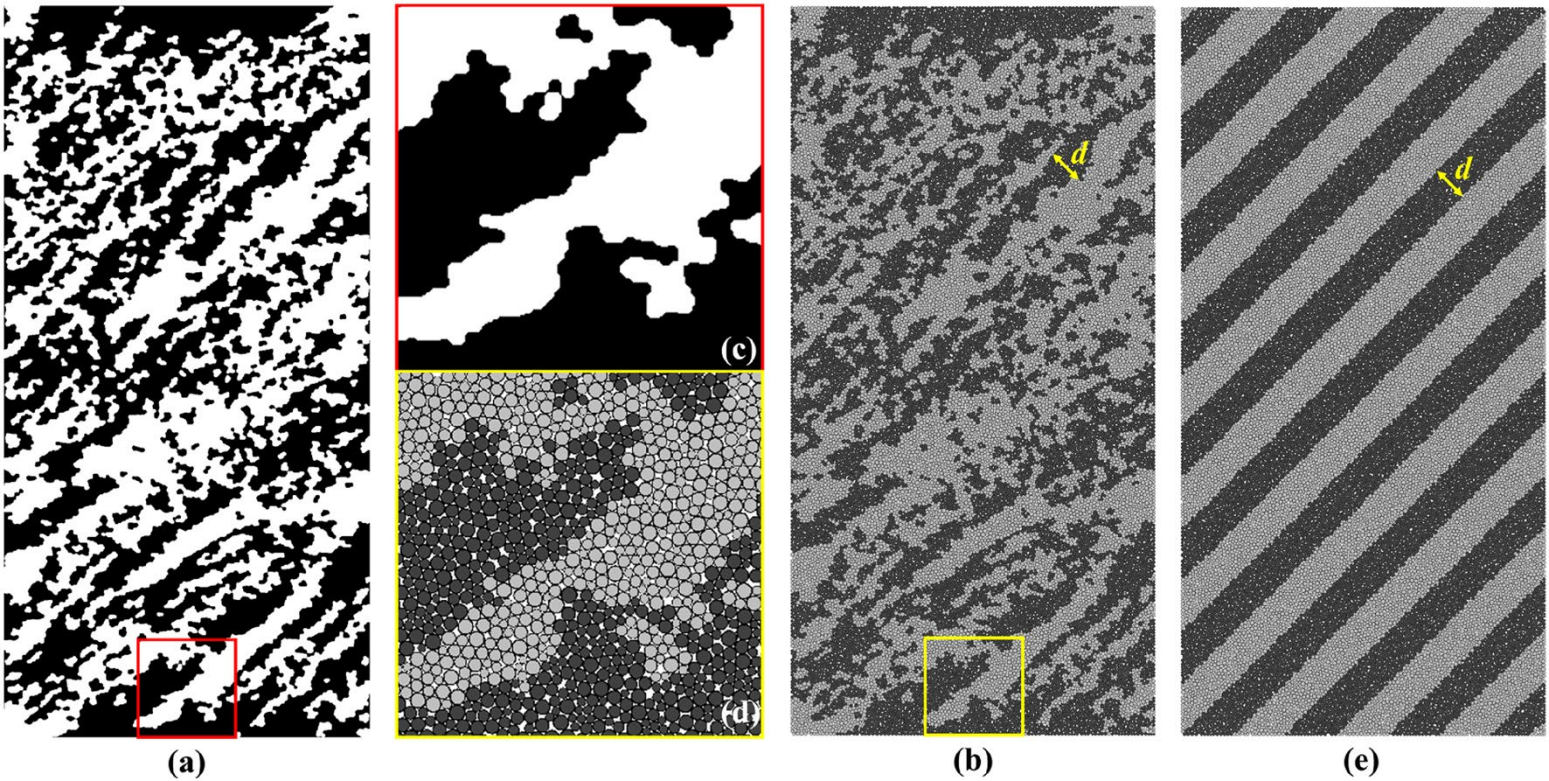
Numerical model of 45° sample, where (a) is the binary CT image of the 45° sample, (b) is the numerical discrete element CT model, (c,d) are the local details of binary image and numerical model, respectively, and (e) is the ideal numerical model with similar layered thickness.
